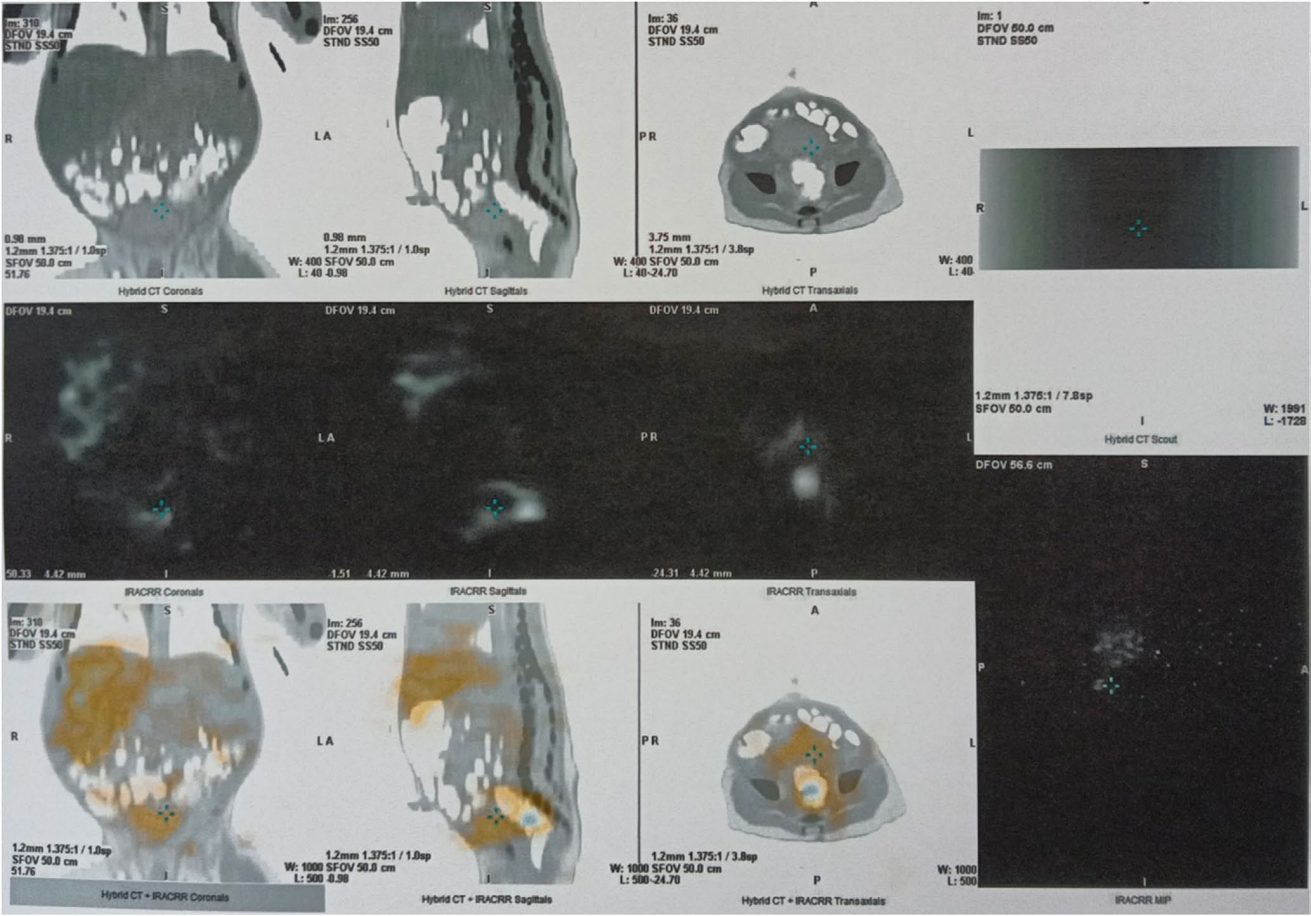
Good and homogenous tracer uptake was seen in the liver with satisfactory pool clearance. Faint tracer accumulation is seen in the lower abdominal region at 24 h of the study, with intraluminal uptake observed in the rectosigmoid colon on SPECT–CT.
